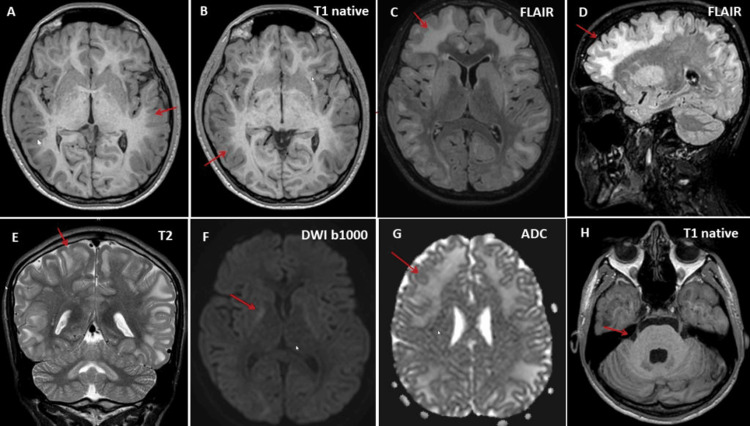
An MRI scan of the brain performed at the age of 18 showed extensive, partially confluent hyperintensities in the subcortical white matter bilaterally on T1, T2, FLAIR, and DWI (panels A-E, G), with a predominance in the frontal lobes and less pronounced in the brainstem (Panel H). These were not visible in the DWI scan (Panel F). FLAIR: fluid-attenuated inversion recovery; DWI: diffusion-weighted imaging
